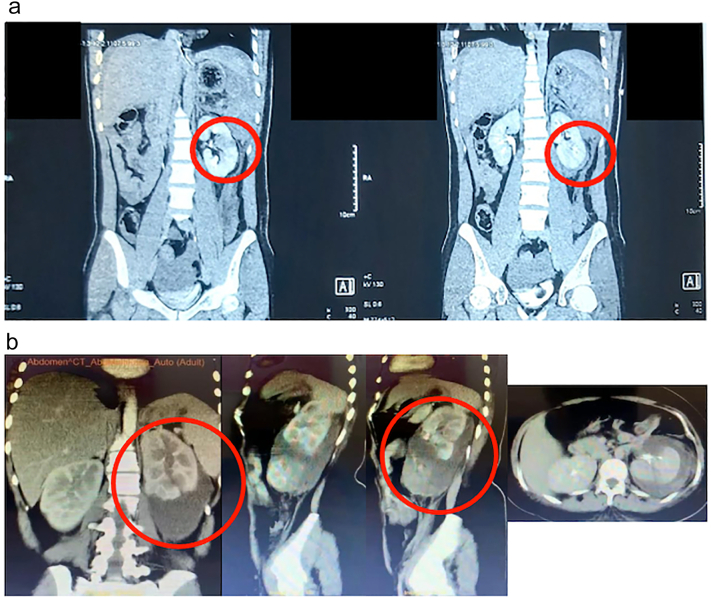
(a). First-time abdominal CT scan result. (b). Second-time abdominal CT scan result.
